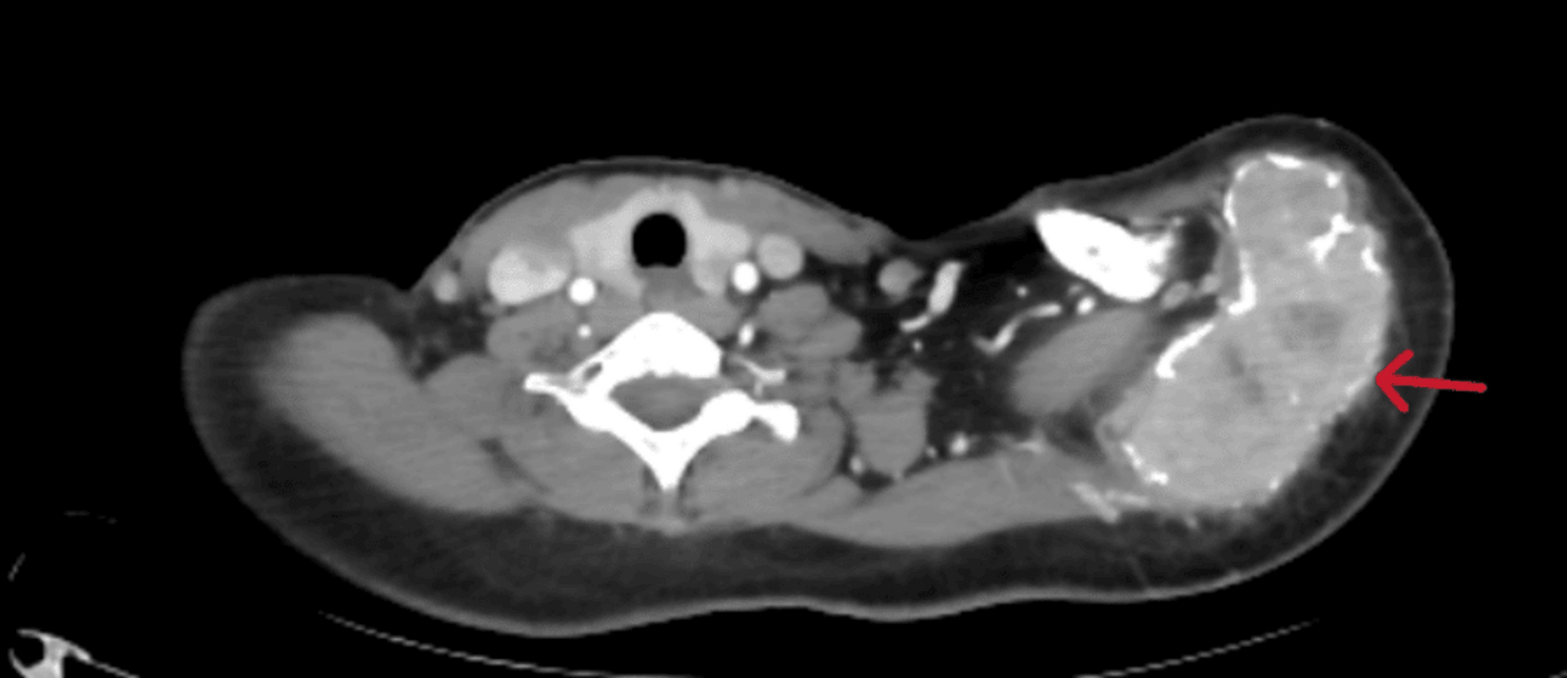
Axial CT scan of the shoulder showing a mass with irregular borders and cortical breakthrough (arrow).
